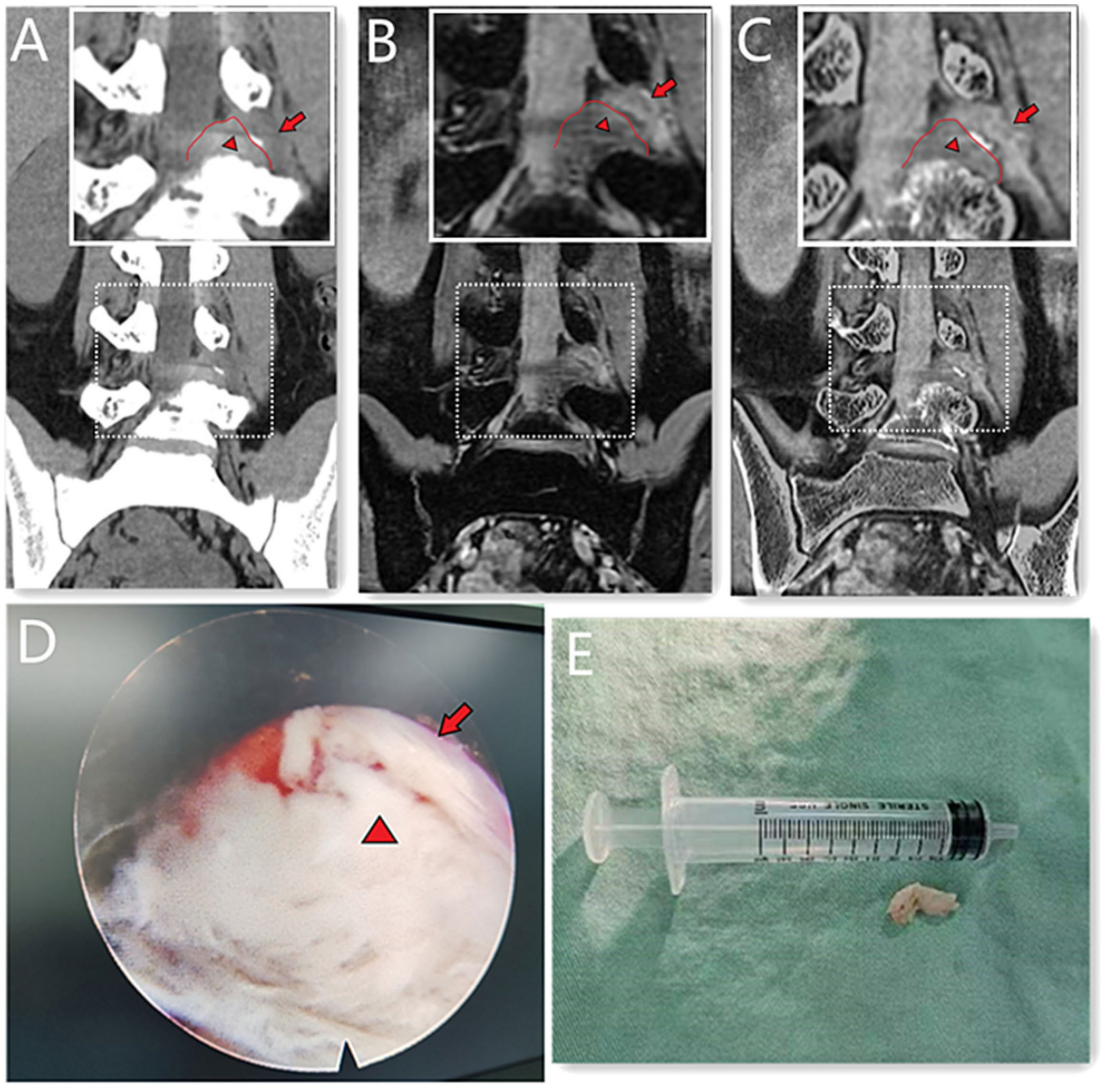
A 39-year-old male with L_4/5_ intervertebral disc herniation, compressing the left nerve root. The red lines indicate the edge of the herniated disc (A–C). The arrow represents the nerve root and the arrow head represents the disc herniation (A–E). The CT image (A) quality scores of bony structure, discs and nerve roots were 5^*^, 3, 3. The 3D MERGE (B) image quality scores were 2, 5 and 4. The image quality scores of CT 3D-MERGE fusion image (C) were 5, 5, and 4. (D) Showed a photo of transforaminal endoscopic lumbar discectomy for L_4/5_ and (E) showed the excised intervertebral disc. *The bone structure score of CT images was 5 points, which was evaluated in the bone window and not represented in figure.
